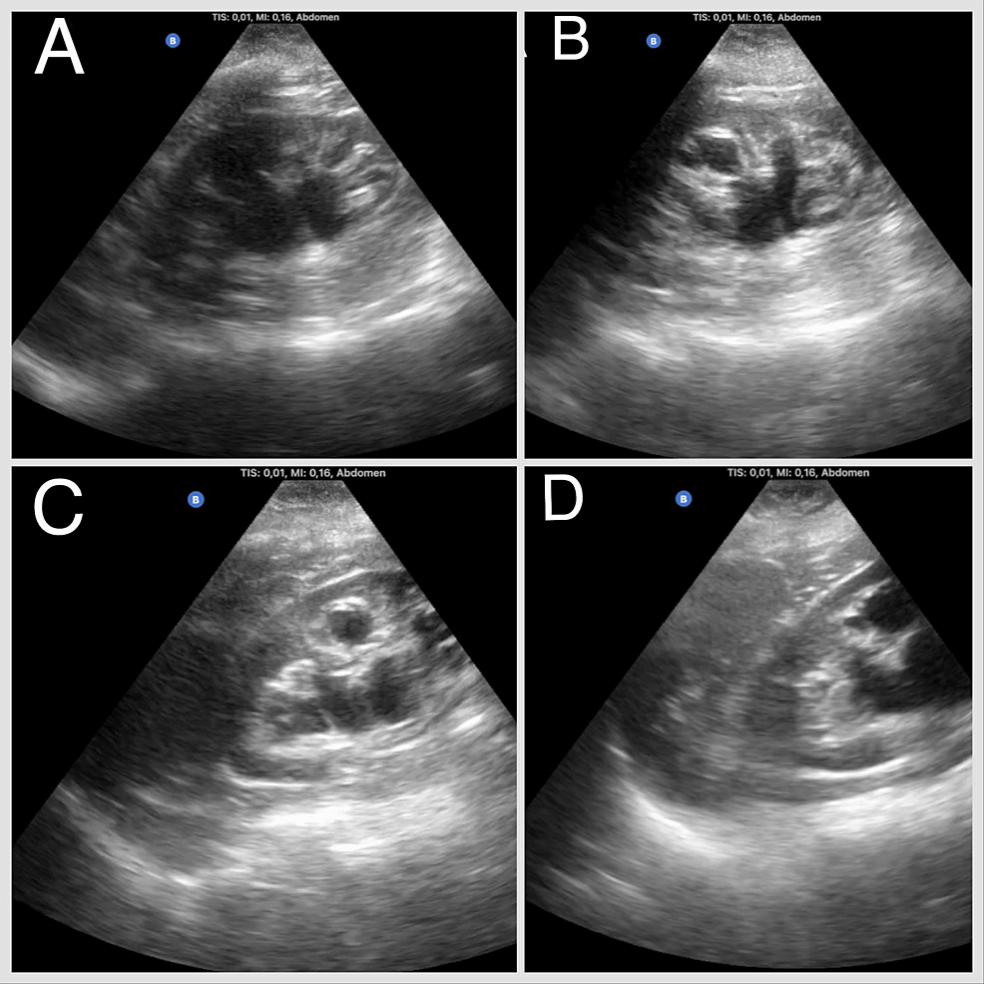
Renal ultrasound: longitudinal plane of the left kidney (A), (B), (C), and (D). Mild-to-moderate pyelocaliectasis of linear appearance without evidence of proximal obstructive factor.
